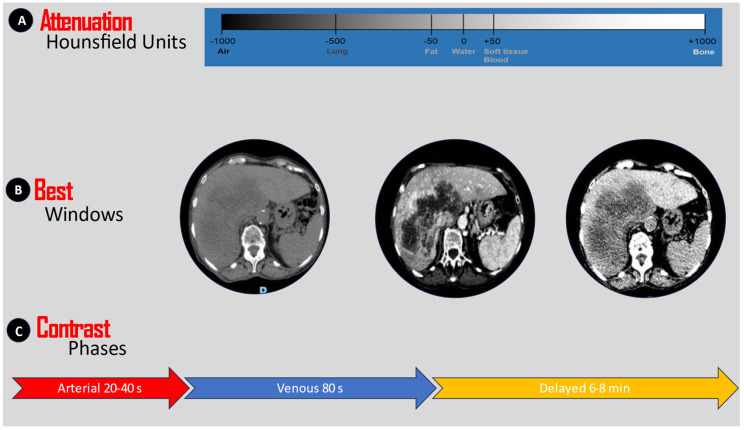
The ABC of lesion enhancement during CT interpretation ((A) attenuation, (B) best window, (C) contrast phases).
